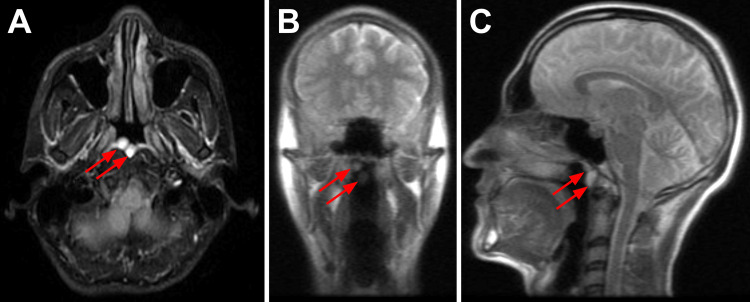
T2-weighted and diffusion-weighted MRI scans of the Tornwaldt cysts T2-weighted fat-suppressed fluid-attenuated inversion recovery image transverse view (A), diffusion-weighted imaging sequences of coronal (B) and sagittal (C) views of magnetic resonance imaging scans showing two hyperintense Tornwaldt cysts (red arrows) each with a diameter of 8 mm, along the posterior wall of the nasopharynx
